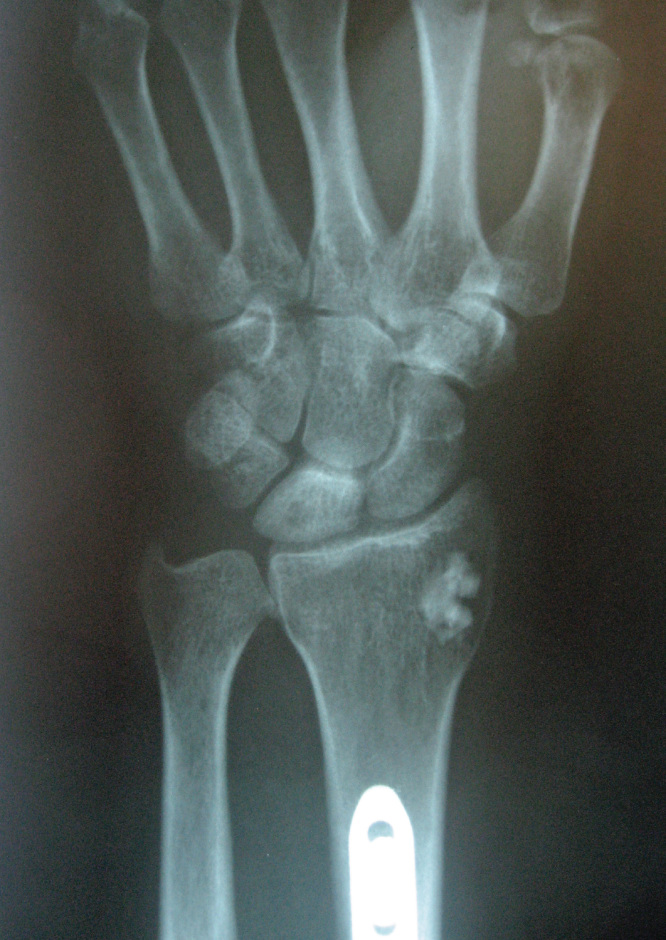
X-ray showing the patient’s wrist after the first operation. Radial shortening and also the donor site of the bone flap can be seen.
